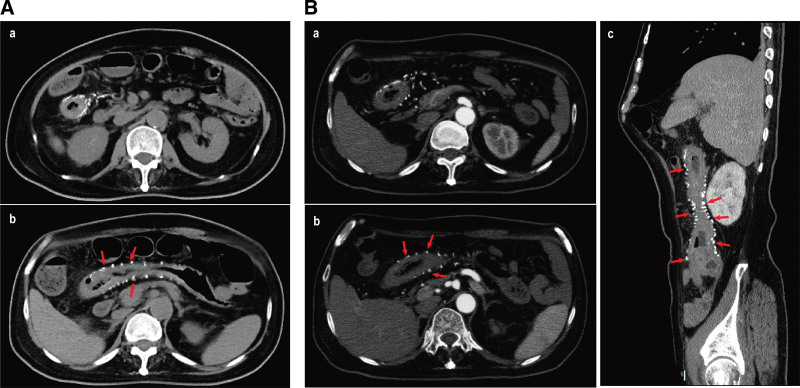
Abdominal CECT and mesenteric vein CTV of the patient with IMP and liver cirrhosis. (A) CECT-depicted thickening of the right hemicolon (A-a), and prominent calcifications of the mesenteric veins along the colonic wall (A-b). (B) CTV showed diffuse colon mural thickening from the ileocecal area to the hepatic flexure colon (B-a), and multiple calcifications were observed in the right colic veins (B-b and B-c). The arrow indicates calcifications. CECT = contrast-enhanced computed tomography, CTV = computed tomography venography, IMP = idiopathic mesenteric phlebosclerosis.
